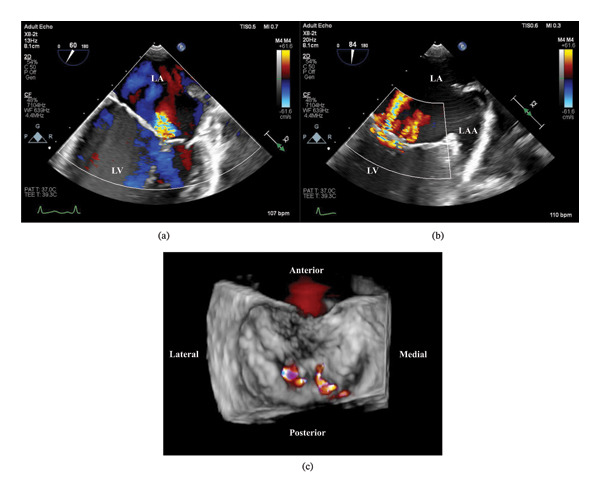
Transesophageal echocardiogram (TEE) images demonstrating diffuse endocardial thickening and valvular thickening, highlighting the mitral valve (MV). (a) Mid‐esophageal (ME) 4‐chamber view of the mitral valve with heterogenous thickening at the tips of the leaflets. Turbulent retrograde flow across the MV visualized. (b) Modified ME 2‐chamber view of the MV and left atrial appendage visualized. Color flow Doppler demonstrating several regurgitant jets across the mitral valve. (c) 3D representation of the mitral valve in a surgical view with applied color flow Doppler. MV thickening is present on the medial portion of the valve, and regurgitant jets are also visualized.
